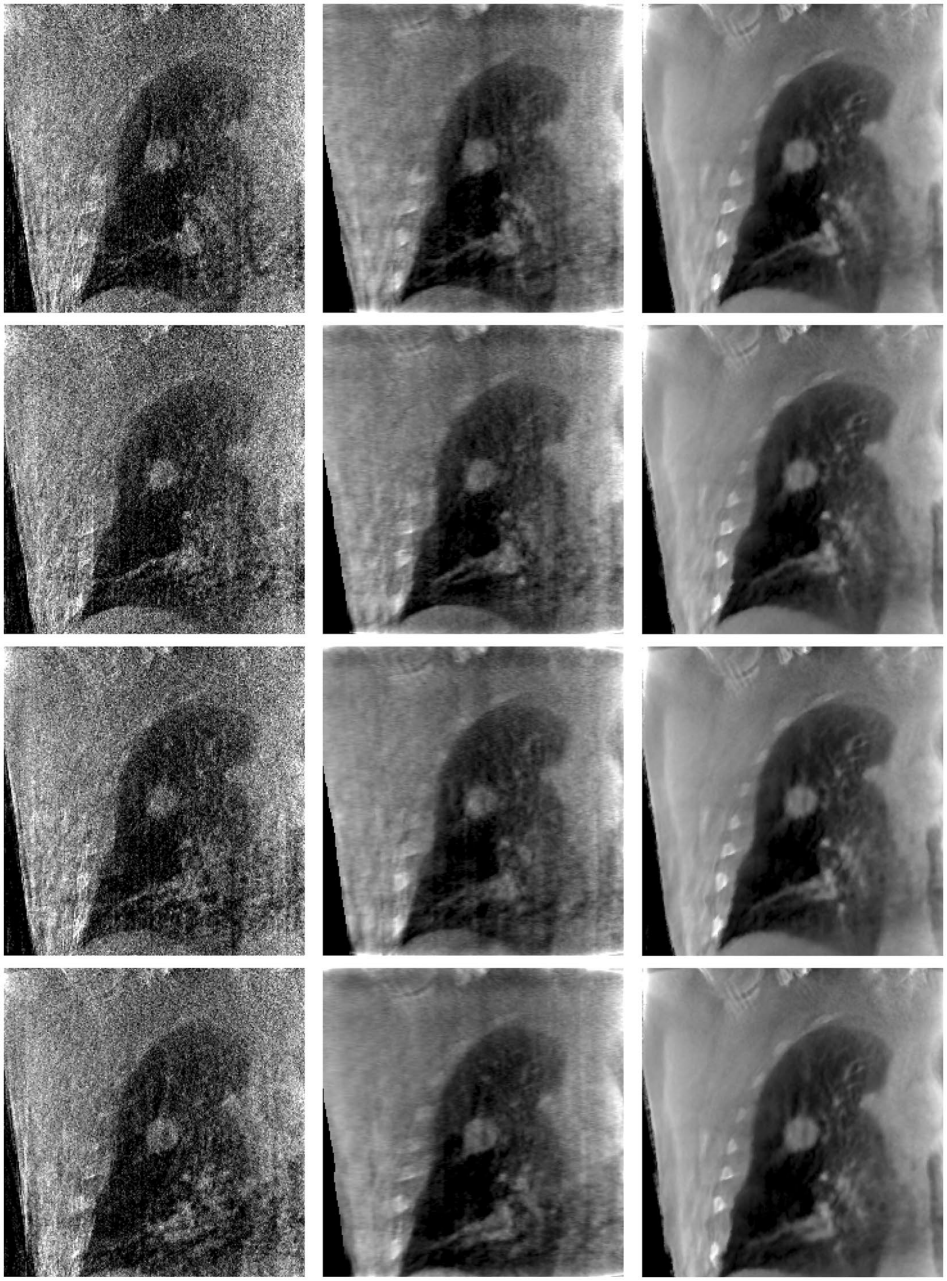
Results of patient data reconstructed by using different methods. The left to the right columns show the 4D-CBCT reconstructed by FDK, SART-TV and proposed MgSS algorithm, respectively.Each row shows images at the different phases in the breathing cycle: 20–30%, 40–50%, 60–70%, 80–90%.
